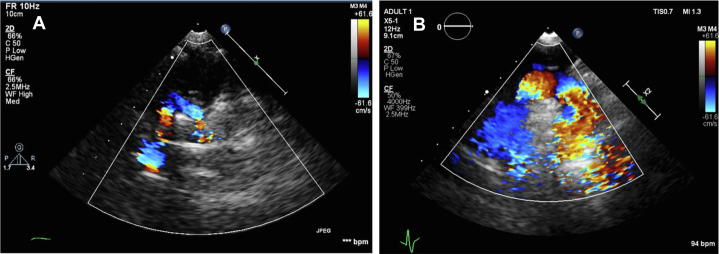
Transthoracic Echocardiography Comparison (A) Preoperative Doppler imaging demonstrating a right-dominant double aortic arch. (B) Postoperative suprasternal long-axis view with color Doppler showing unobstructed flow through the ascending aorta, aortic arch, and descending aorta.
